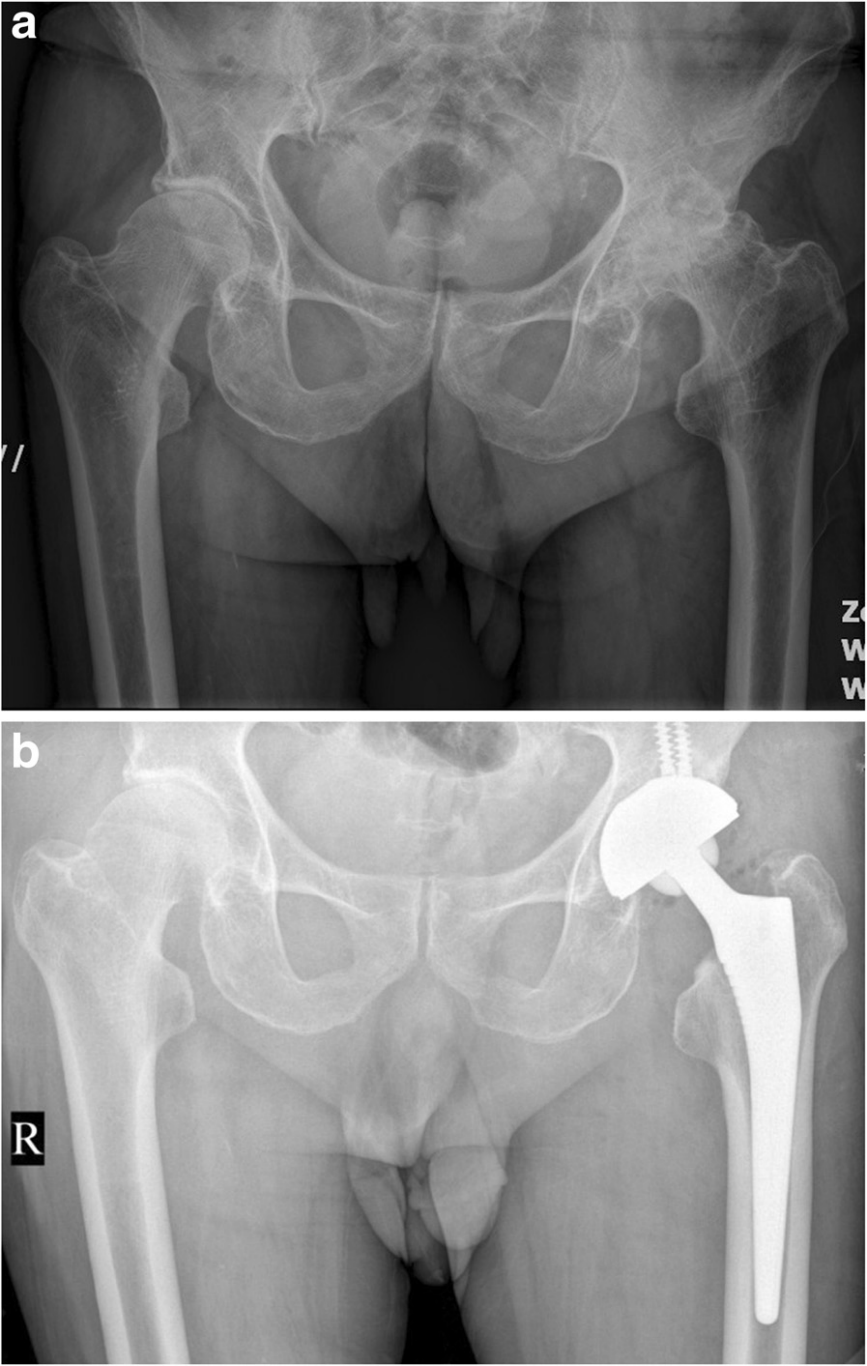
a A preoperative radiograph of the hip of a 71-year-old man who had left hip osteoarthritis. b A radiograph at 44 months after implantation of conventional cementless femoral stem showed solid fixation in a satisfactory position
